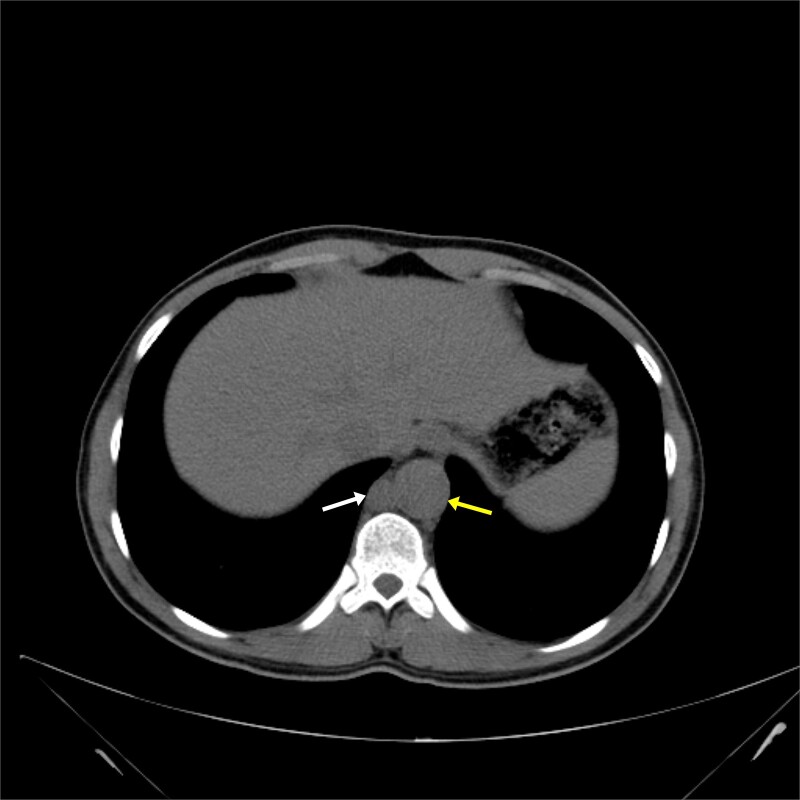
Native chest CT axial section shows mildly dilated thoracic descending aorta measures 3 cm in diameter “yellow arrow,” also inflammatory lymph node 1.1 cm in short axis diameter adjacent to aorta “white arrow”.
